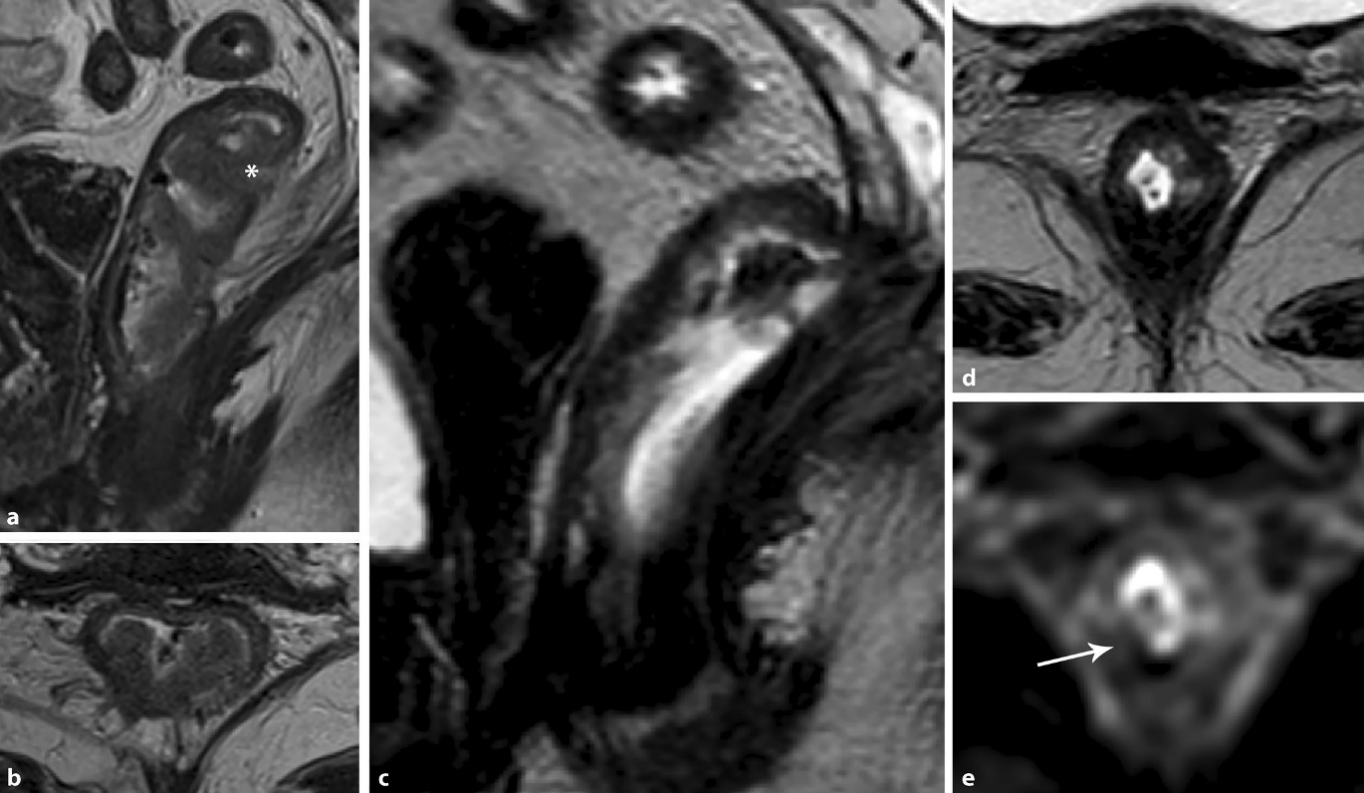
Magnetic resonance imaging (MRI) of a female patient before (a, b) and after chemoradiotherapy (c, d) shows a locally advanced distal rectal cancer (*white arrow*) that responded excellently to neoadjuvant chemoradiotherapy, with a complete response. On restaging MRI with diffusion-weighted imaging, no restricted diffusion was visible (e)
